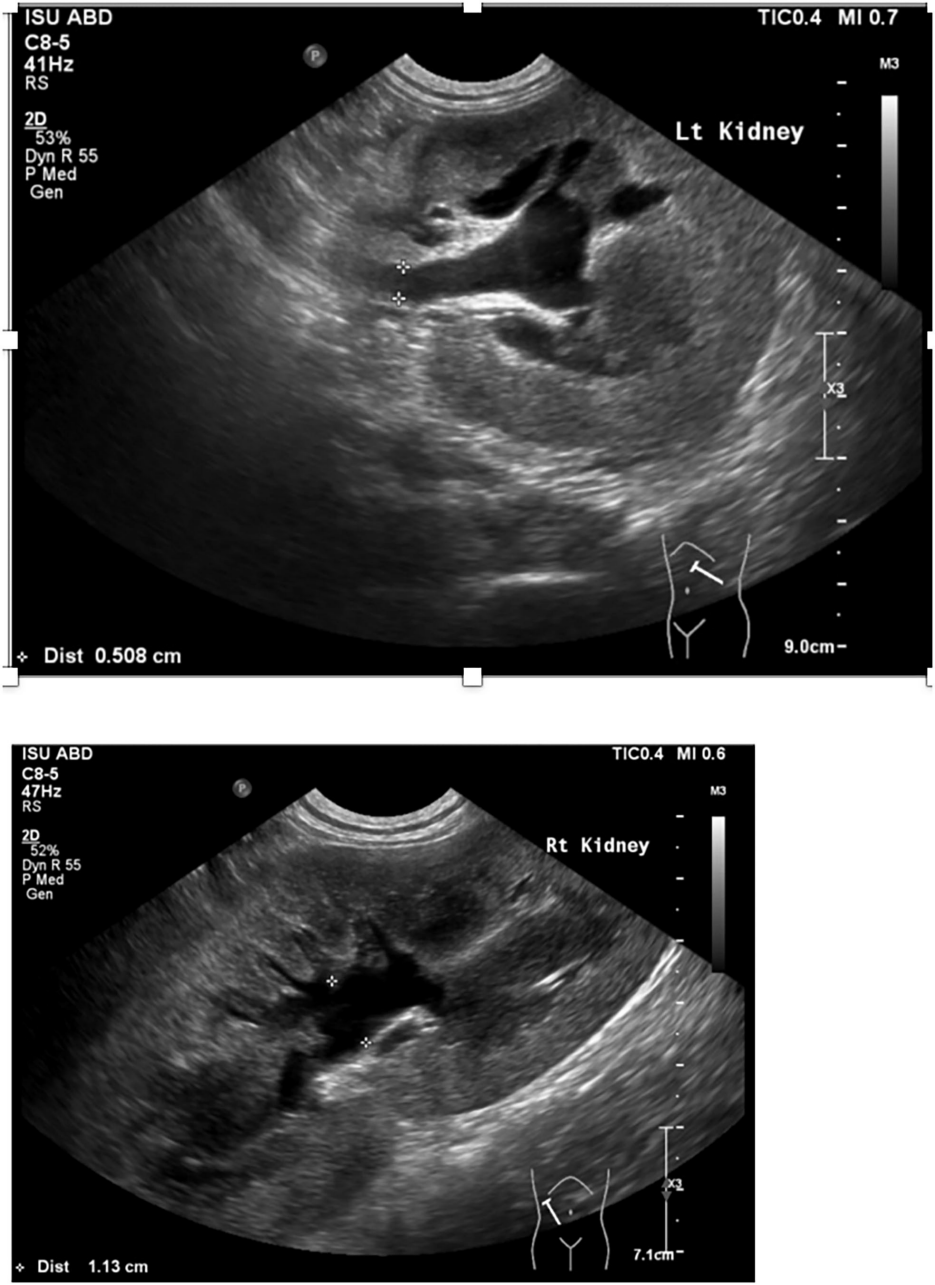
Abdominal ultrasound image of left and right kidney and proximal ureter including pelvic dilation and ureteral dilation.
